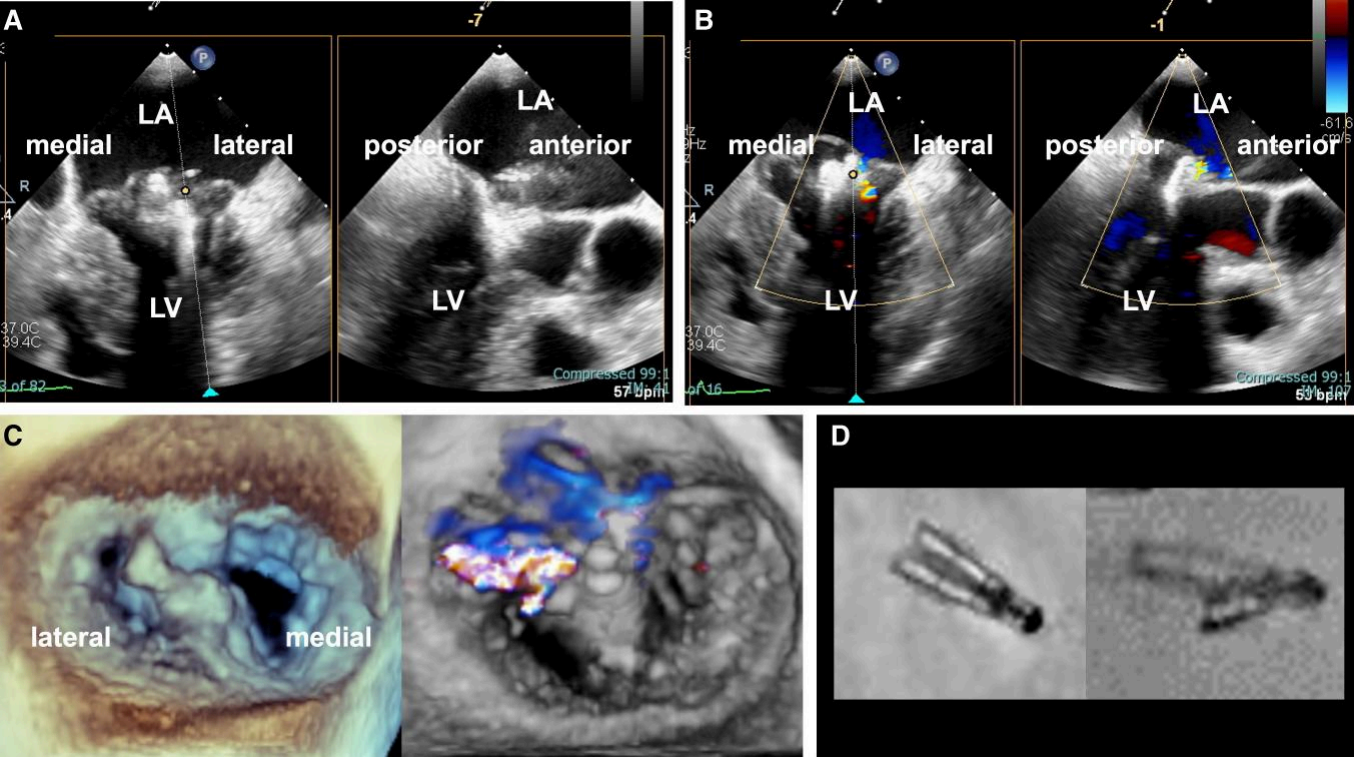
Intra-procedural transoesophageal echocardiogram images of the second transcatheter edge-to-edge mitral valve repair and fluoroscopic images of XTW MitraClip of Patient 2. An additional MitraClip G4 XT system is inserted lateral to the original XTW system (*A*). No residual mitral regurgitation was observed immediately after XT system deployment (*B*). The final three-dimensional transoesophageal echocardiogram demonstrated rigid coaptation, and slight residual mitral regurgitation was observed (*C*). Fluoroscopic images of the XTW MitraClip just after deployment (*D*, left panel), and openings between the clips were visible (*D*, right panel). LA, left atrium; LV, left ventricle.
